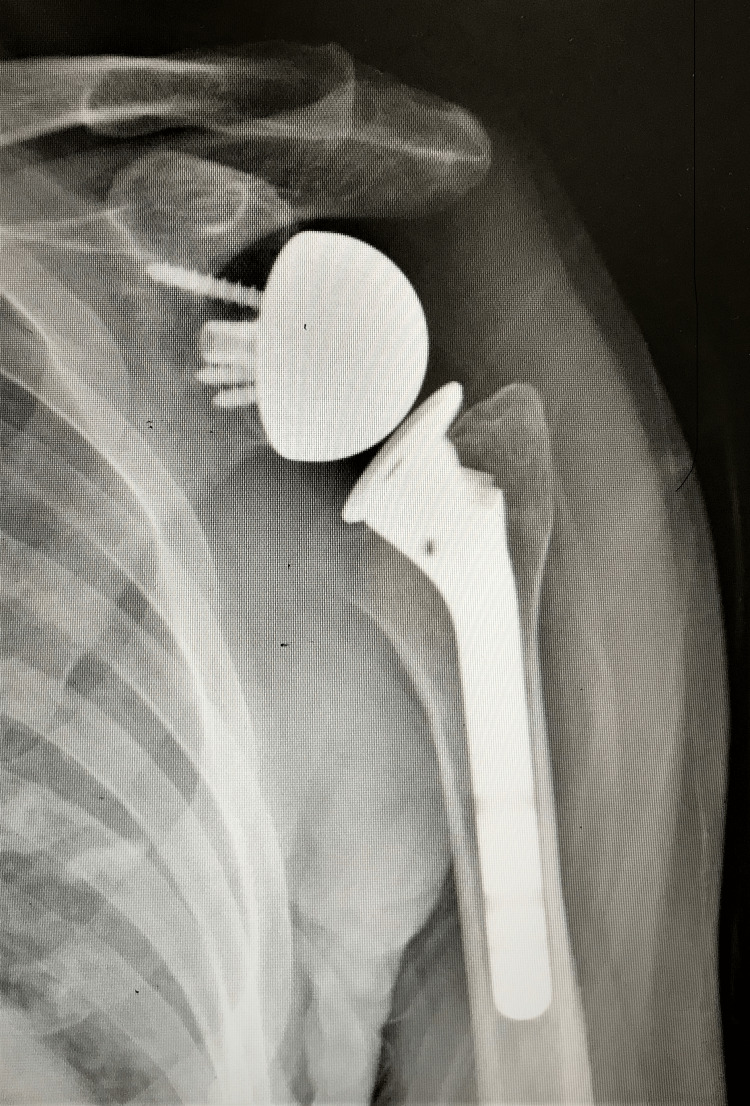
Postoperative 12th-month X-ray of a patient included in the study.
